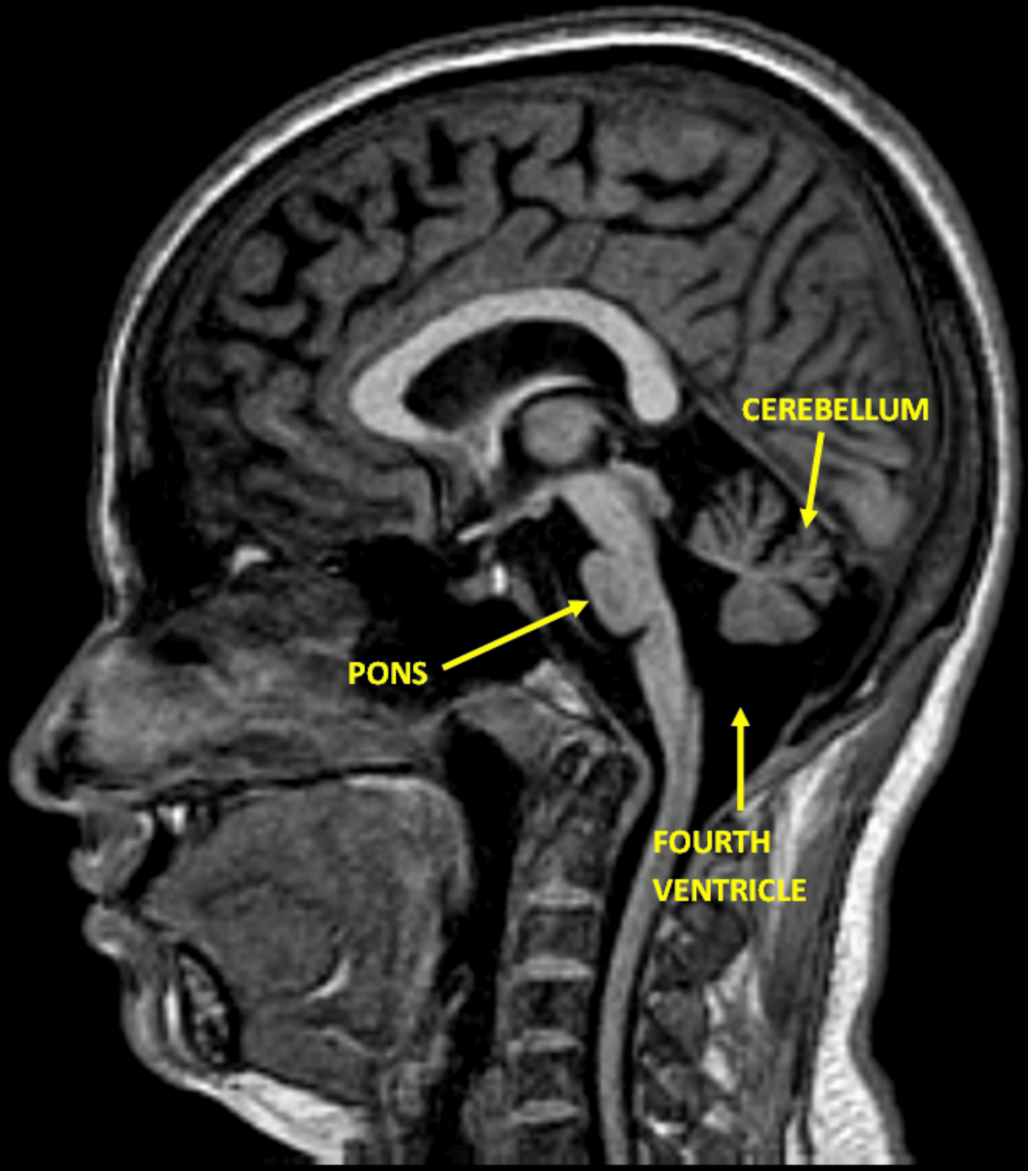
A sagittal T1-weighted image provides a side view of the brain, including the cerebellum, brainstem, and cerebral cortex. There is significant cerebellar atrophy, especially involving the vermis and the anterior lobe. The pons also appears atrophic, with a flattened ventral surface. The fourth ventricle is prominently dilated due to the atrophy. This image is crucial for assessing the extent of atrophy and the involvement of the brainstem structures.
